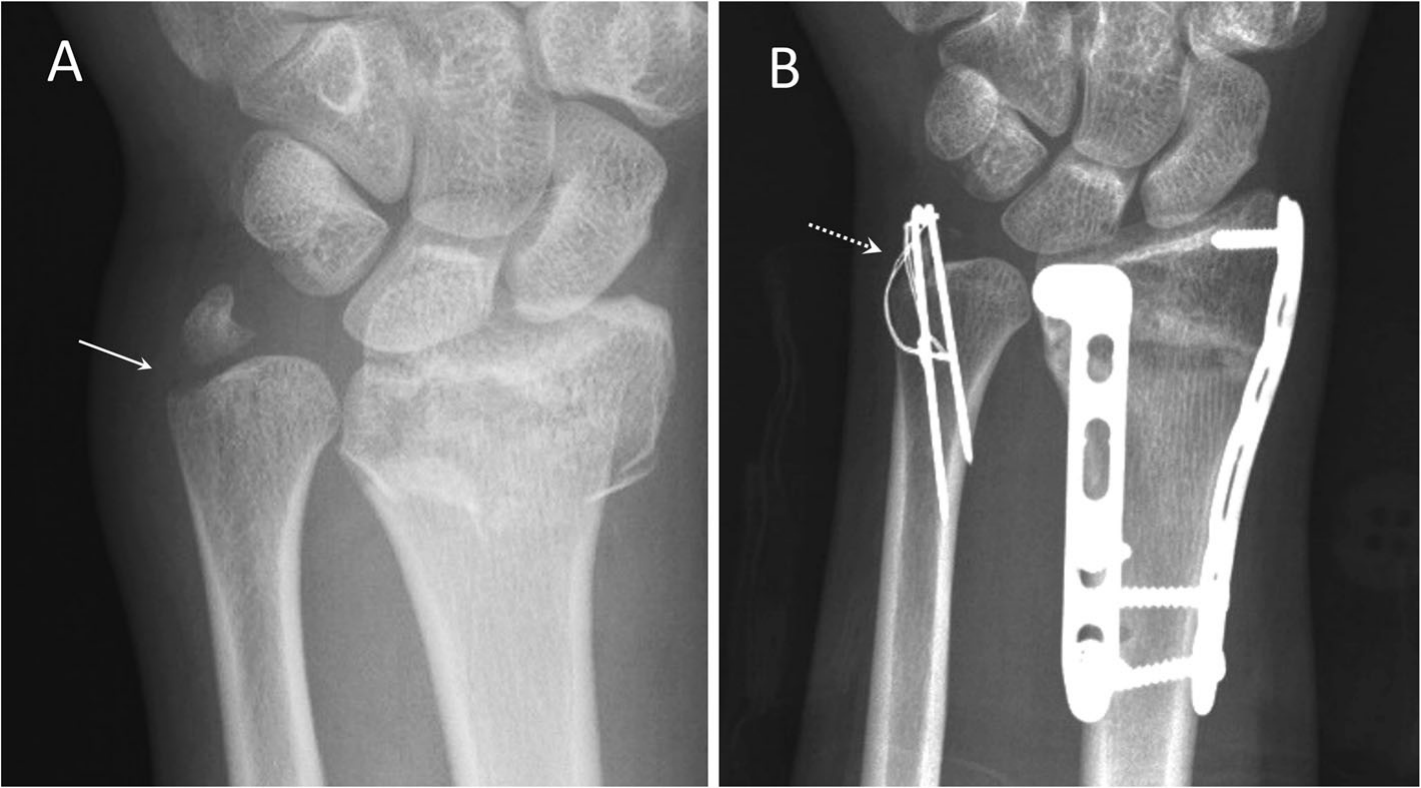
Posteroanterior radiographs of a 20-year-old female patient with distal radioulnar fractures. a Displaced ulnar styloid base fracture (arrow). b Osseous union (dotted arrow) at 3 months after surgical fixation with tension band wire
